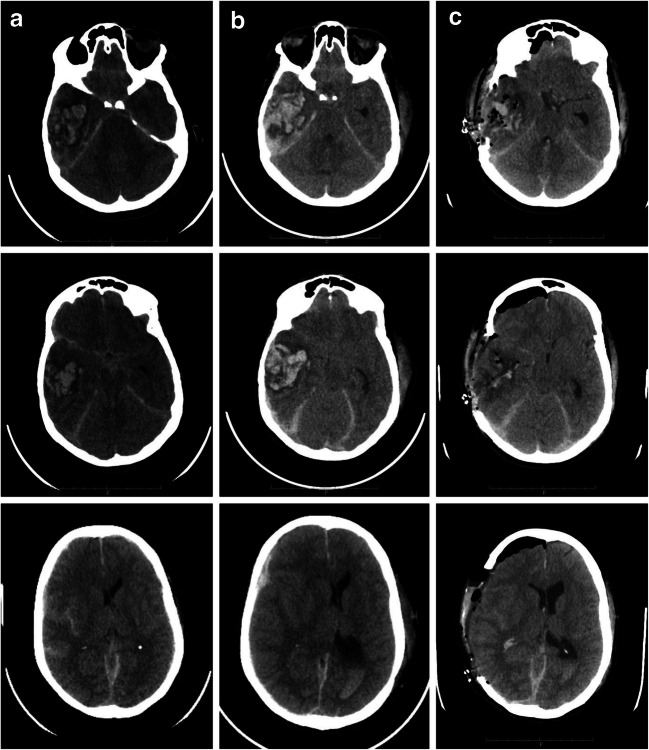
CT scan brain trauma survey for traumatic brain injury in LMS case. a From top to bottom, different slices of CT scan at the admission to ED department. b Same slices from top to bottom, registered at 6 h from the first CT scan; it could be noted an increase and a better consolidation of the intraparenchymal hematoma in the right temporal lobe; furthermore, an intraventricular hemorrhage and a worsening of the midline shift toward the left hemisphere are shown. c Postoperative CT scan; the different slices show the result of craniectomy and right temporal ICH evacuation, with significant reduction of midline shift and an initial reappearance of basal cisterns
